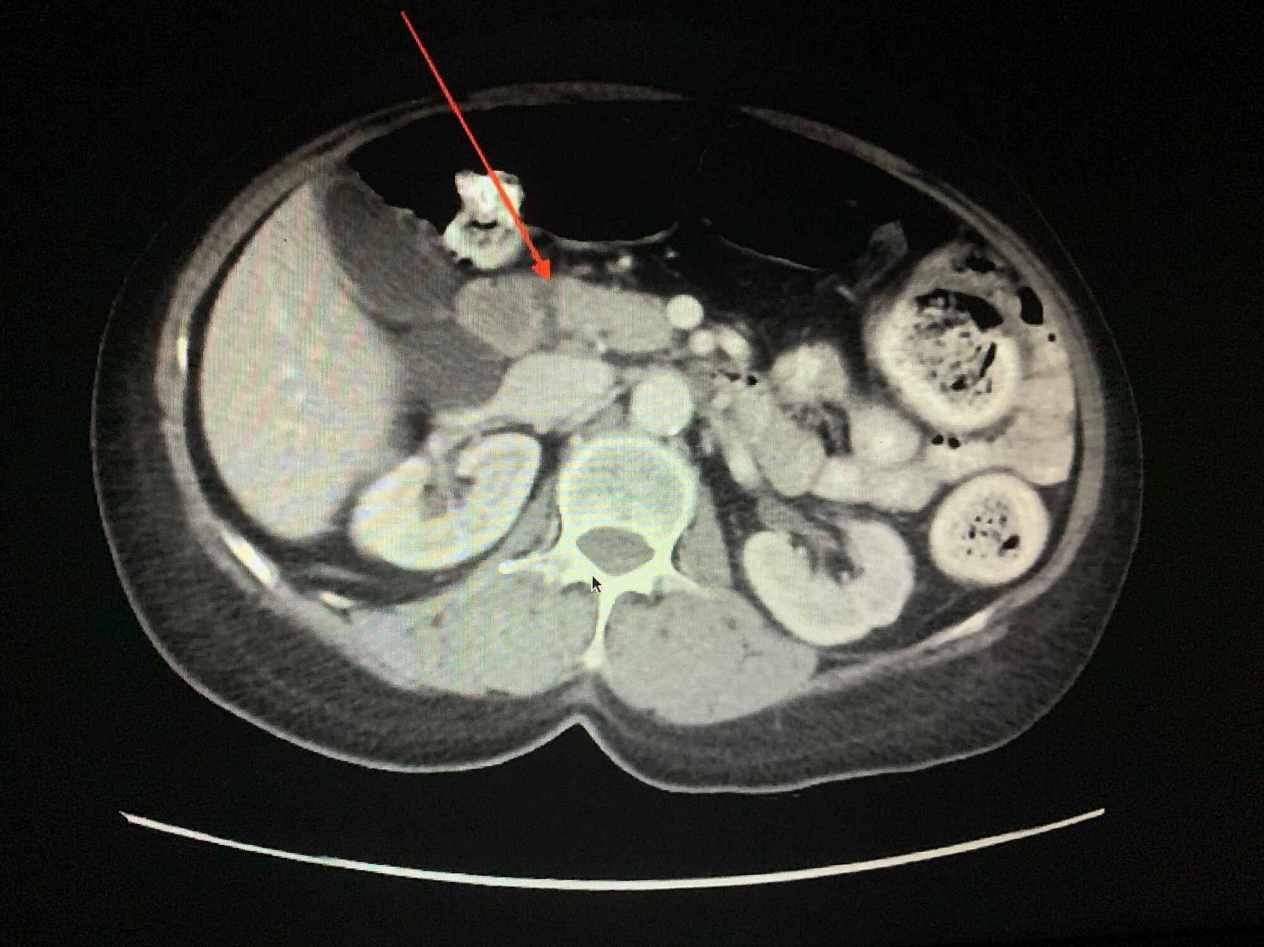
CT of the abdomen and pelvis demonstrating fat infiltration within the pancreaticoduodenal groove consistent with acute interstitial pancreatitis
